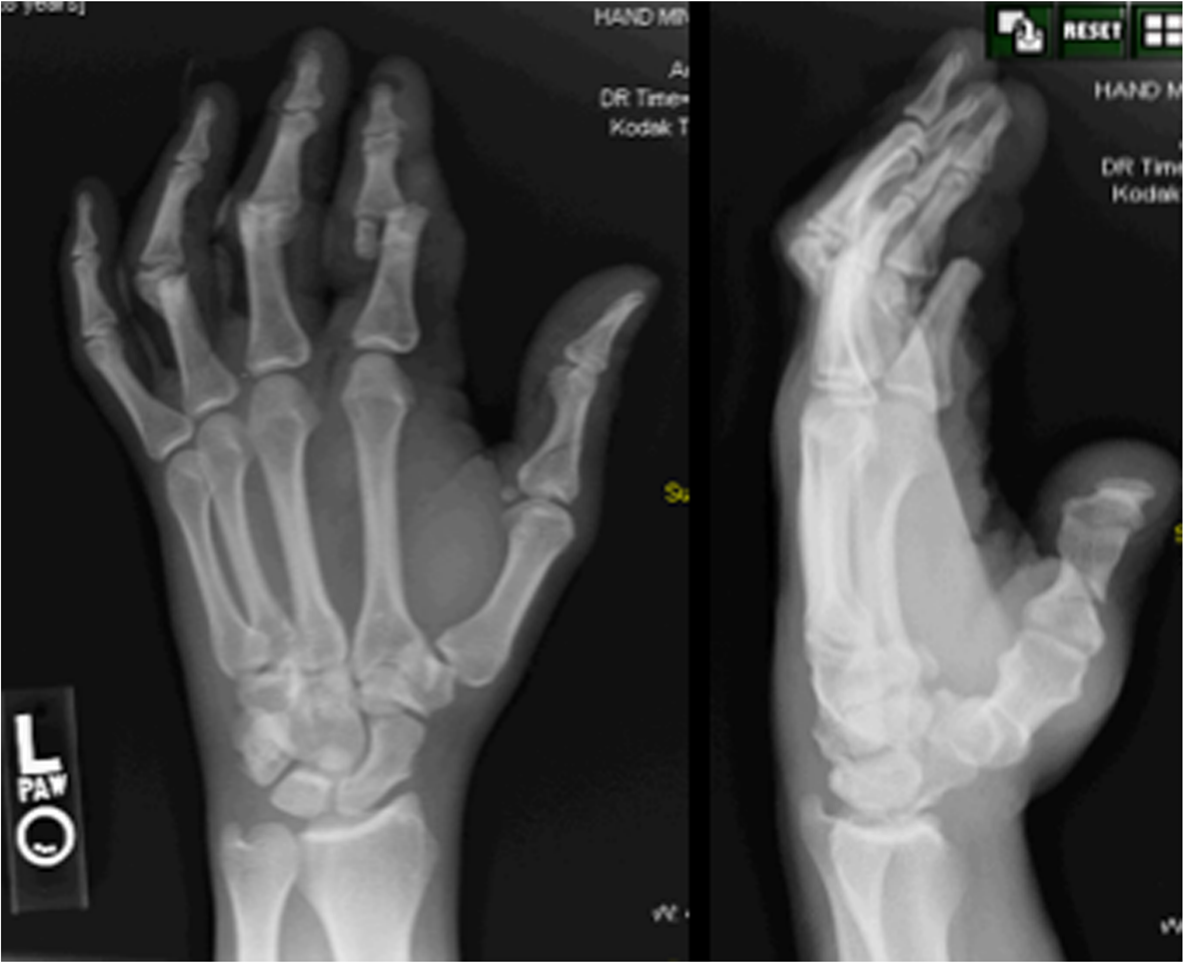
Preoperative radiographs showing proximal interphalangial joint fracture dislocations of left index, long, and ring fingers and proximal phalanx fracture of the thumb.
